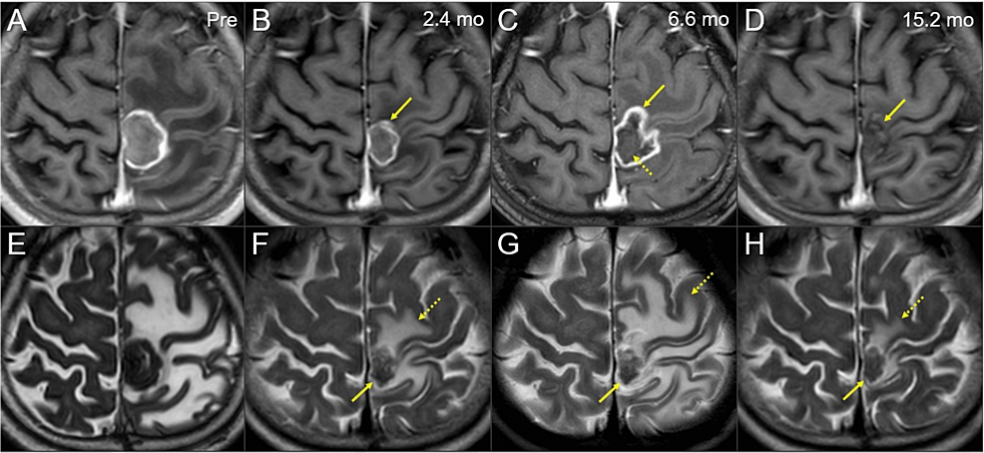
Magnetic resonance images before and after the five-fraction stereotactic radiosurgery The images show axial CE-T1-WIs (A-D); axial T2-WIs (E-H); before SRS (A, E); 2.4 months (mo) after the initiation of SRS (B, F); at 6.6 months (C, G); and at 15.2 months (D, H). (A-H) These images are shown at the same magnification and coordinates under co-registration and fusions. (B, F) The enhancing lesion (arrow in B) shrank with a decrease in the enhancement. The corresponding T2-mass (arrow in F) shrank, its intensity generally increased and became more heterogeneous, and its configuration became more irregular. The perilesional edema (dashed arrow in F) decreased remarkably. (C, G) The enhancing lesion (arrow in C) with peripheral dominance expanded remarkably and was irregular in shape, avoiding the cortex and extending into the white matter. Meanwhile, the T2-mass (arrow in G) remained regressed and its shape and intensity were the same. However, the perilesional edema (dashed arrow in G) expanded to the same degree as before SRS. Upon closer inspection, the mass lesion (dashed arrow in C) corresponding to the T2-mass was visible inside the enhancing lesion. (D, H) The lesion (arrow in D) became almost non-enhanced, and only a slightly low-intensity mass corresponding to the T2 mass was visualized. The T2-mass (arrow in H) maintained its size, and the perilesional edema (dashed arrow in H) was remarkably reduced and its degree was minimal. The images at 12.3 months (not shown) after the SRS initiation (5.2 months after BEV administration) already showed the same degree of improvement as at 15.2 months. (G, H) No apparent signal changes were observed in the contra-lateral para-central sulcus gyri. CE: contrast-enhanced; WIs: weighted images; SRS: stereotactic radiosurgery; BEV: bevacizumab
